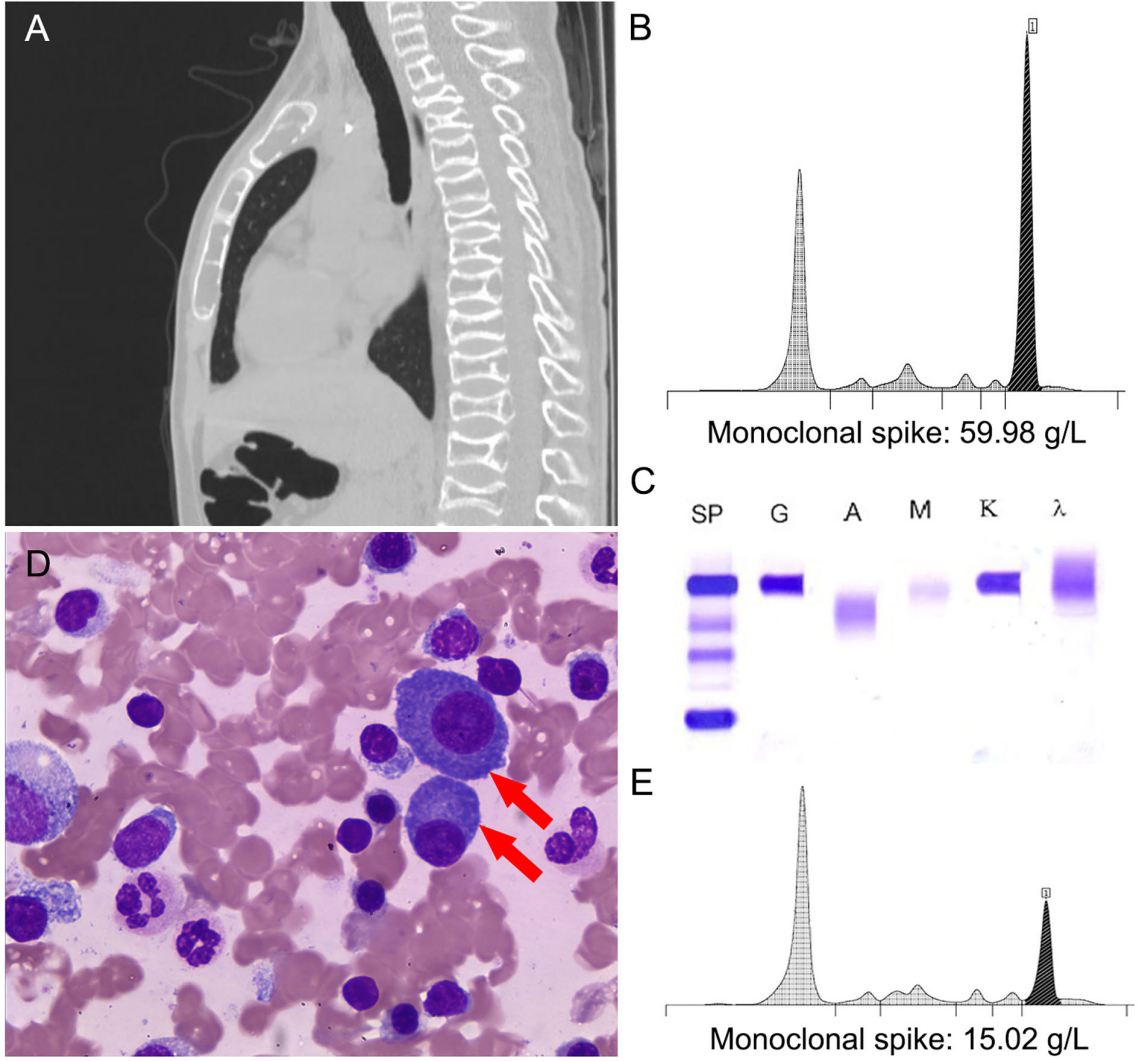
(A) Chest computed tomography (CT) scan. (B) Protein electrophoresis for gamma globulin levels (black) before treatment. (C) Serum immunofixation electrophoresis demonstrating monoclonal IgG kappa proteins. (D) Bone marrow cytology (red arrows: plasma cells, ×400). (E) Protein electrophoresis for gamma globulin levels (black) after autologous hematopoietic stem cell transplantation (ASCT).
